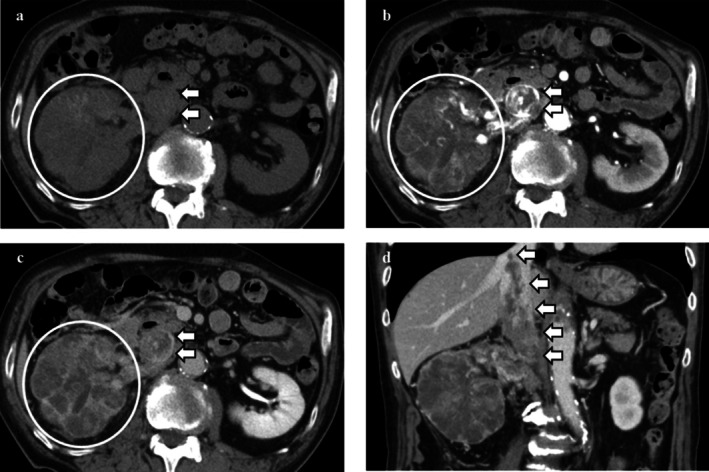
Dynamic abdominal CT at initial presentation. Dynamic abdominal CT at initial presentation revealed a large, heterogeneously enhancing renal mass measuring 91 mm in the right kidney. Focal areas of the mass showed strong enhancement in the corticomedullary phase with washout in the nephrographic phase, consistent with the typical contrast‐enhancement pattern of clear cell RCC. A tumor thrombus extending into the IVC up to the level of the hepatic veins was also observed. (a) Axial view: Non‐contrast phase; (b) Axial view: Corticomedullary phase; (c) Axial view: Nephrographic phase; (d) Coronal view: Corticomedullary phase. White circles indicate the renal mass, and white arrows indicate the tumor thrombus within the IVC.
